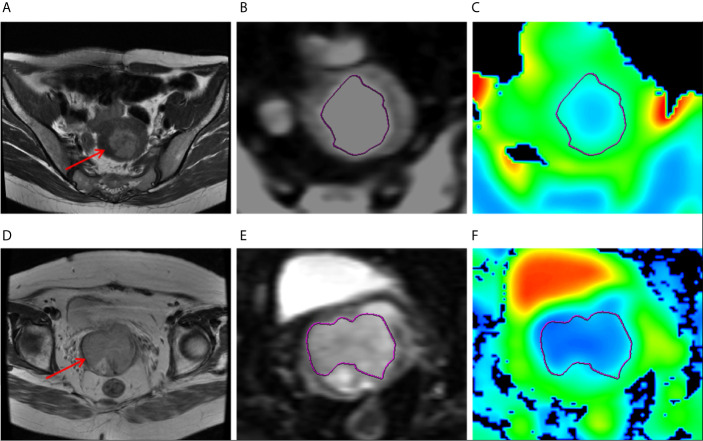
(A–C) MR images of a 47-year-old woman with grade 1 endometrioid carcinoma, pathologically proven DMI and absence of LVSI or LNM. (A) Axial T2-weighted image showed that there was an irregular mass in the uterine cavity (arrow), the tumor size 3.5 cm × 3.5 cm. (B) ROI at the maximum area of the lesion on DWI. (C) The ADC map. (D–F) MR images of a 47-year-old woman with grade 1 endometrioid carcinoma, pathologically proven DMI, and LVSI but absence of LNM. (D) Axial T2-weighted image showed that there was an irregular mass in the uterine cavity (arrow), the tumor size 3.5 cm × 4 cm. (E) ROI at the maximum area of the lesion on DWI. (F) The ADC map.
